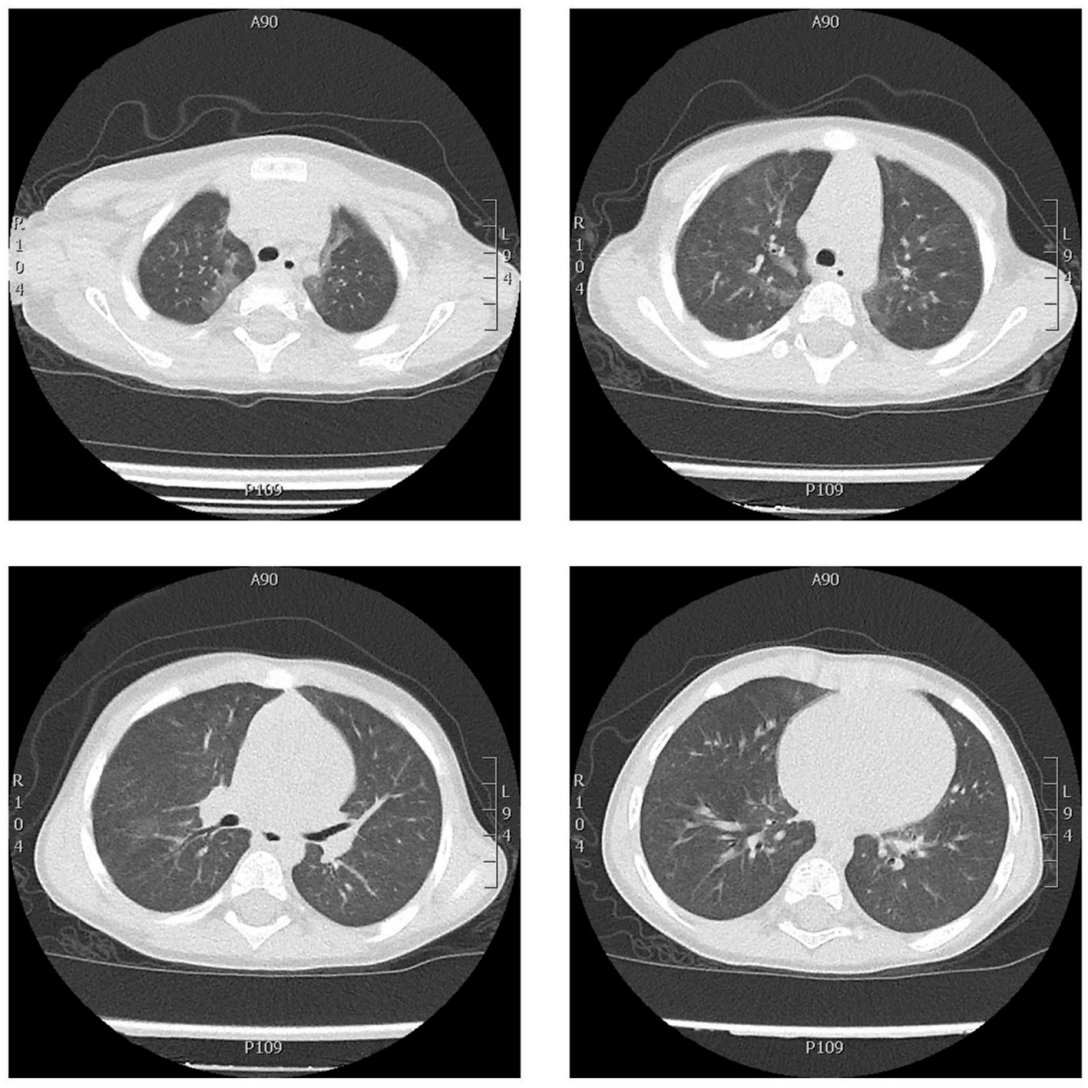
Chest CT axial scan showing ground-glass thickening in both lungs, more evident in the upper and the lower left lobes, less extended and more nuanced than the CT scan performed 6 months before. No signs of interstitial fibrosis.
